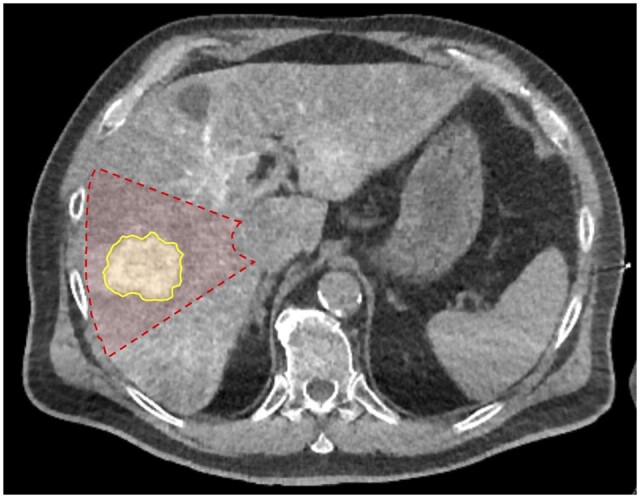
Volume measurements of peripherally located tumours were overestimated by the Couinaud anatomic model when selective angiography was performed (branch of the anterior division of the right hepatic artery in this case). Shown is a 5.5-cm HCC tumour of segments 5/8 and its corresponding tumoural angiosome, as seen on Angio-CT (yellow area; Patient 21 from Supplementary Table S1). The Couinaud segment is shown in red.
